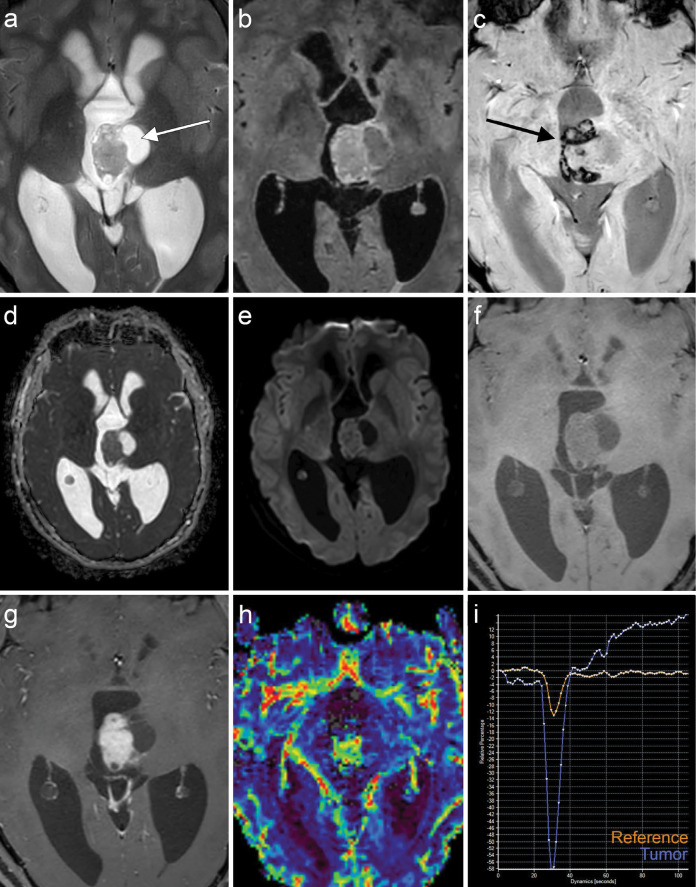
Magnetic resonance images (MRI) of the *EWSR1::PATZ1* gene fusion tumor in the third ventricle. (a) T2 weighted images (T2‑WI) and (b) fluid attenuated inversion recovery (FLAIR) images show the multicystic mass, with incomplete suppression of the content of the cysts (arrow) on T2‑FLAIR. (c) Susceptibility‑weighted imaging (SWI) shows central and peripheral signal loss caused by calcifications and hemosiderin deposition (arrow). Apparent diffusion coefficient maps (d) and b1000 diffusion‑weighted images (e) show that the tumor exhibited facilitated diffusion compared with the gray matter with T2 shine through. T1‑WI before (f) and after (g) gadolinium contrast injection reveals intense contrast enhancement. Dynamic susceptibility contrast perfusion map (h) and curve (i) confirm relative hyperperfusion of the tumor (blue line) compared with normal white matter (orange line) with contrast leakage.
